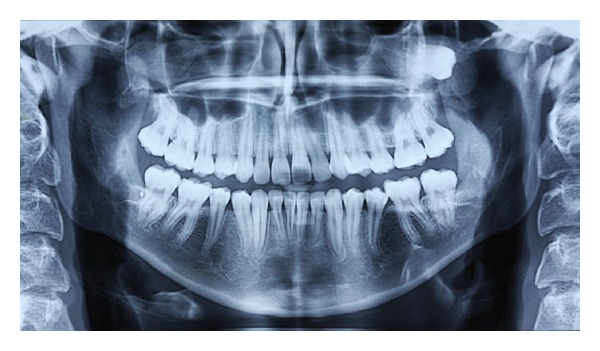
A panoramic radiograph revealed that the left maxillary third molar was displaced in a posterosuperior direction.
